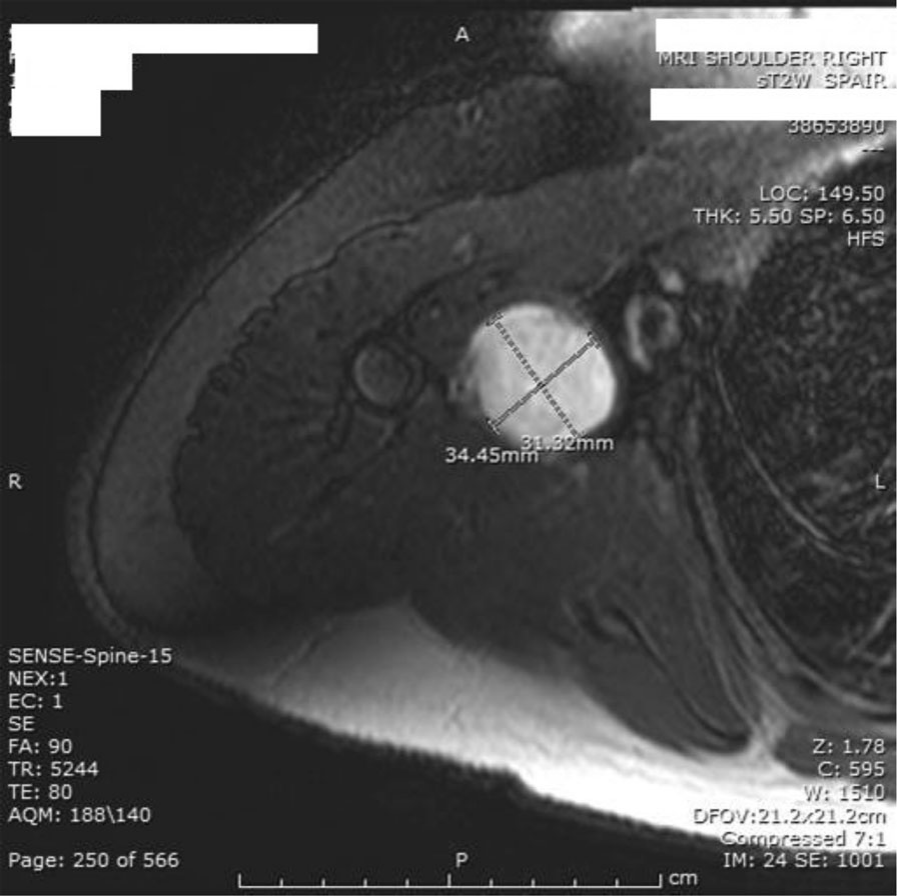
MRI (axial view) showing 3 × 3 cm mass displacing the axillary artery
